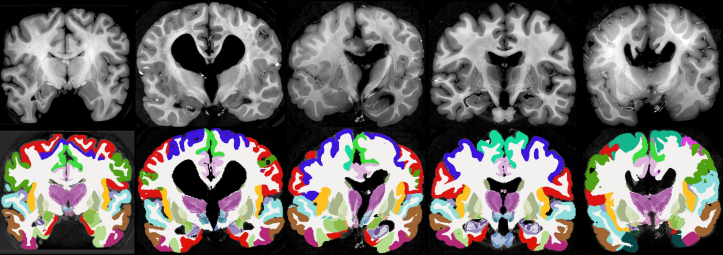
Coronal slices of the five HiP-CT scans (top) and their corresponding automated segmentations using NextBrain (bottom).
